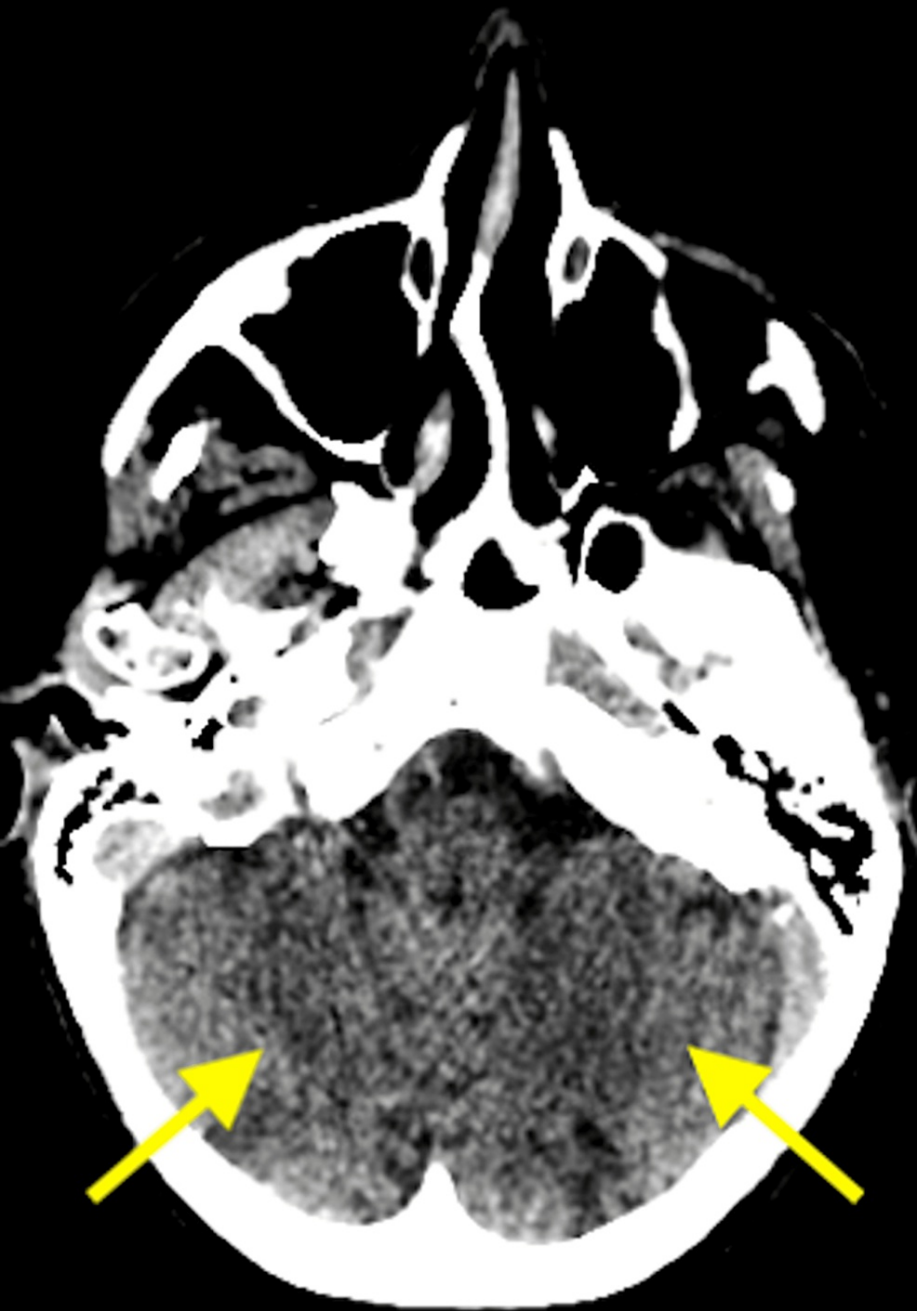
An axial CT head scan showing acute bilateral superior cerebellar infarcts (arrows).
